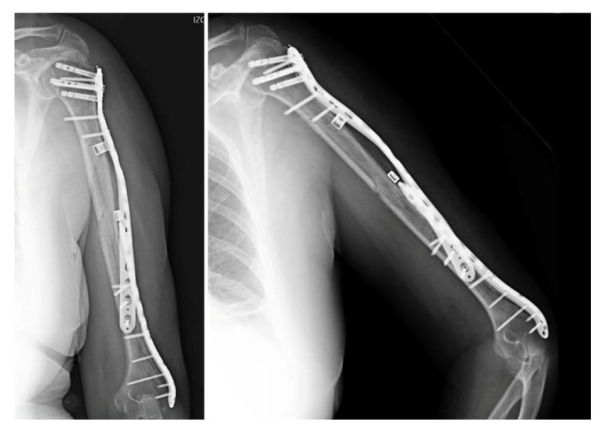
Radiographic follow-up at 6 months post-op.
